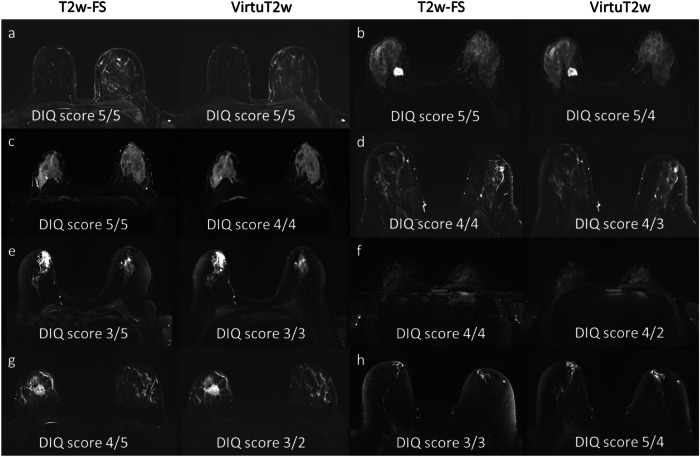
Example of image cases with DIQ ratings given by the two readers. The scores above each image indicate the respective reader’s answers, with Reader 1 presented on the left side and Reader 2 on the right side. In most cases (a–e), the DIQ was higher for the original T2-weighted fat-saturated (T2w-FS) images than for the virtual T2w-FS (VirtuT2w) images. An exception is in h, in which the fat saturation did not work as intended, and the DIQ of the T2w-FS was rated by both readers as only acceptable. In addition, two VirtuT2w images (f, g) were rated by Reader 2 as having a poor DIQ (score 2). No images in the whole cohort were rated as having a very poor quality. DIQ, Diagnostic image quality
